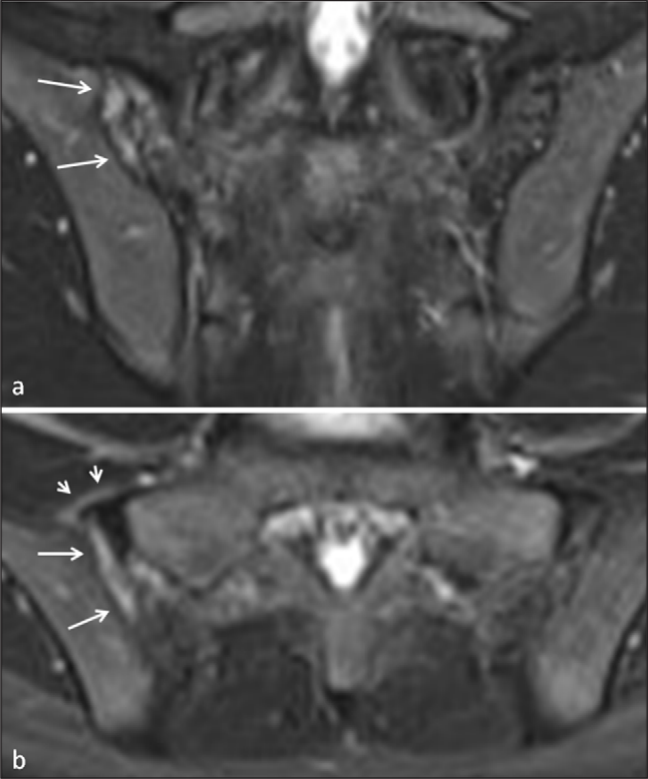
a. Semicoronal and b. axial STIR MR image in a 12-year-old boy with enthesitis-related arthritis demonstrates enthesitis of the retroarticular interosseous ligaments on the right side (arrows). Note also the sacroiliitis with fluid and capsulitis at the right SI joint (short arrows).
